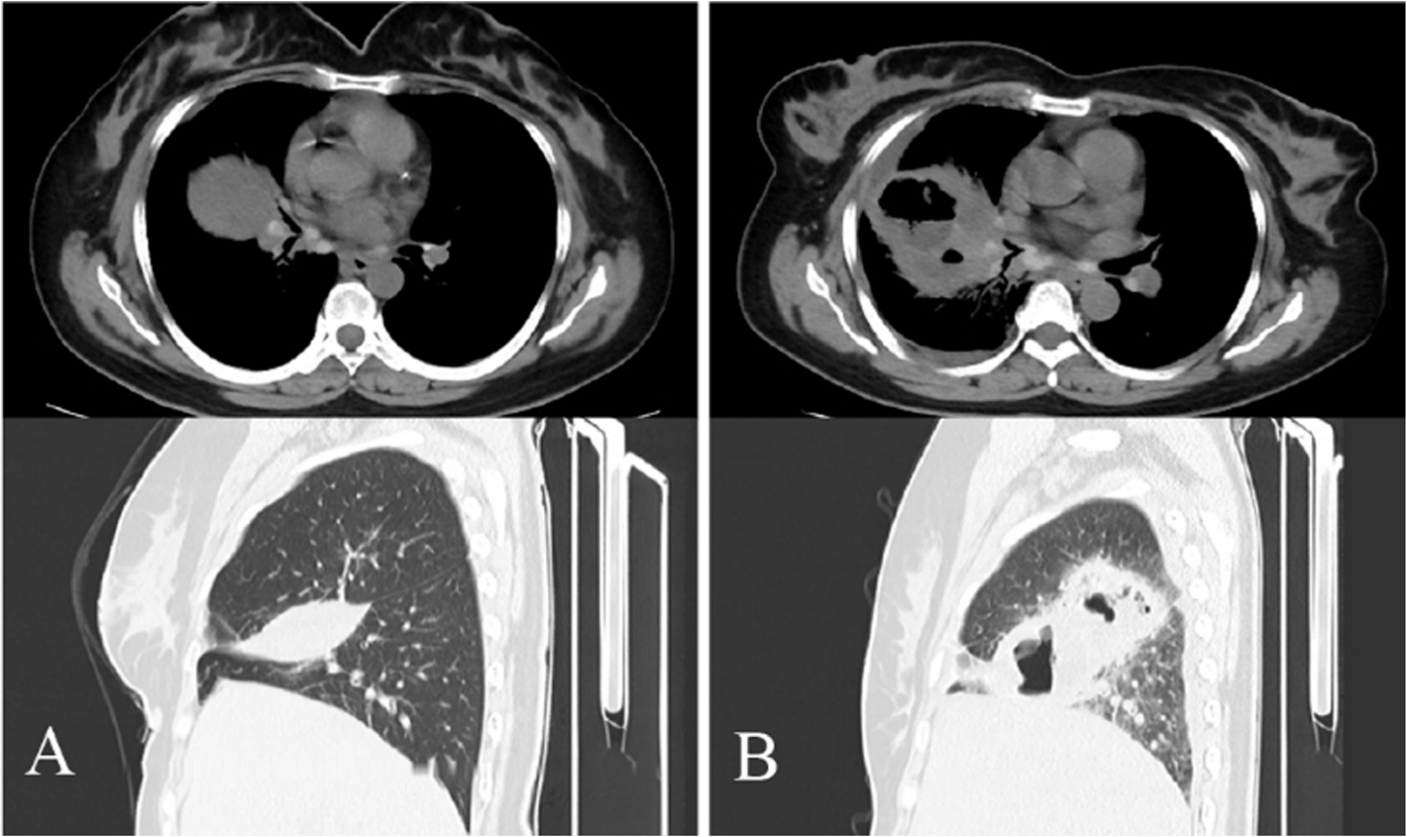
CT showed oblique fissure effusion of right lung on Oct. 8 (a) and showed huge thick-walled cavity in the right lung on Oct. 31 (b)
